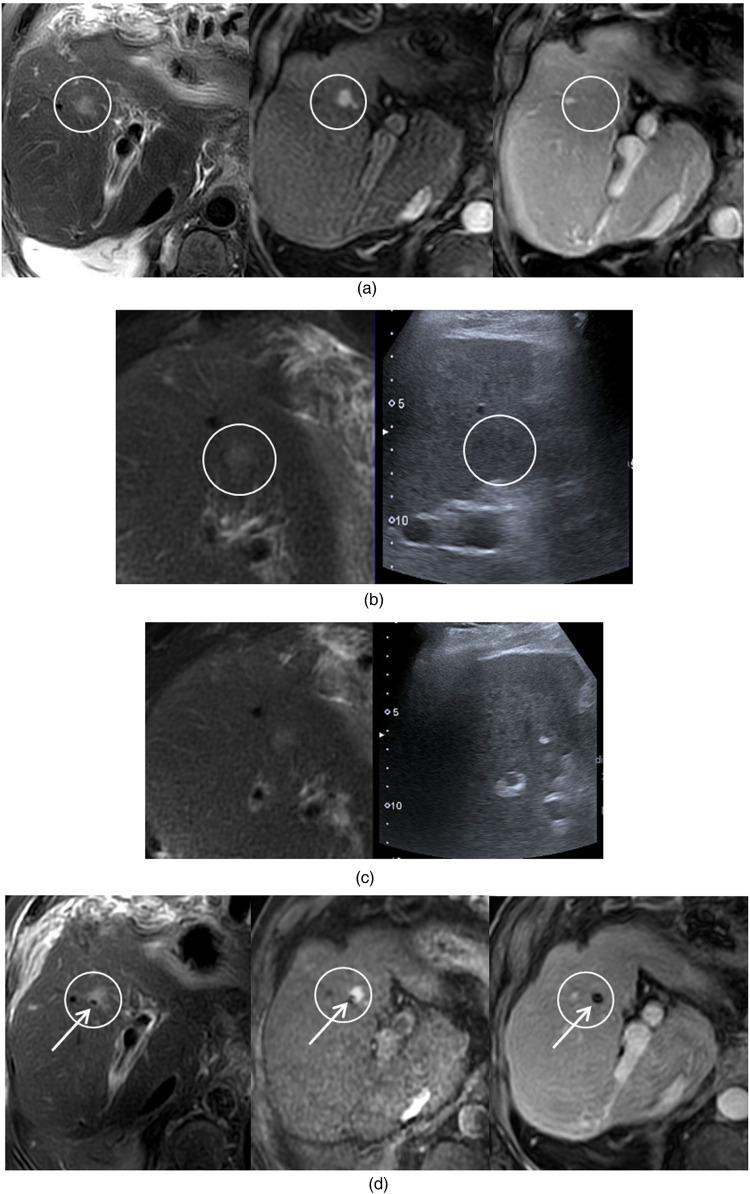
Cirrhotic patient with HCC scheduled for thermal ablation. A second small nodule was identified in the right liver during pre-procedural imaging. The objective was to localize this second nodule for percutaneous treatment during the same session. Lesion detection: (a) An 8 mm nodule is visualized on T2-weighted and T1 arterial-phase MRI, but not on T1 portal-phase imaging. (b) Fusion imaging was performed with T2 MRI. Due to anterior bowel interposition, an oblique intercostal approach was required. The nodule appeared as a slightly hypoechoic focus on ultrasound, although without certainty. Lesion localization: (c) The fiducial marker (white arrow) was placed under combined ultrasound and MR T2 fusion guidance. (d) Immediate post-procedural MRI confirmed the appropriate positioning of the marker in close contact with the nodule.
